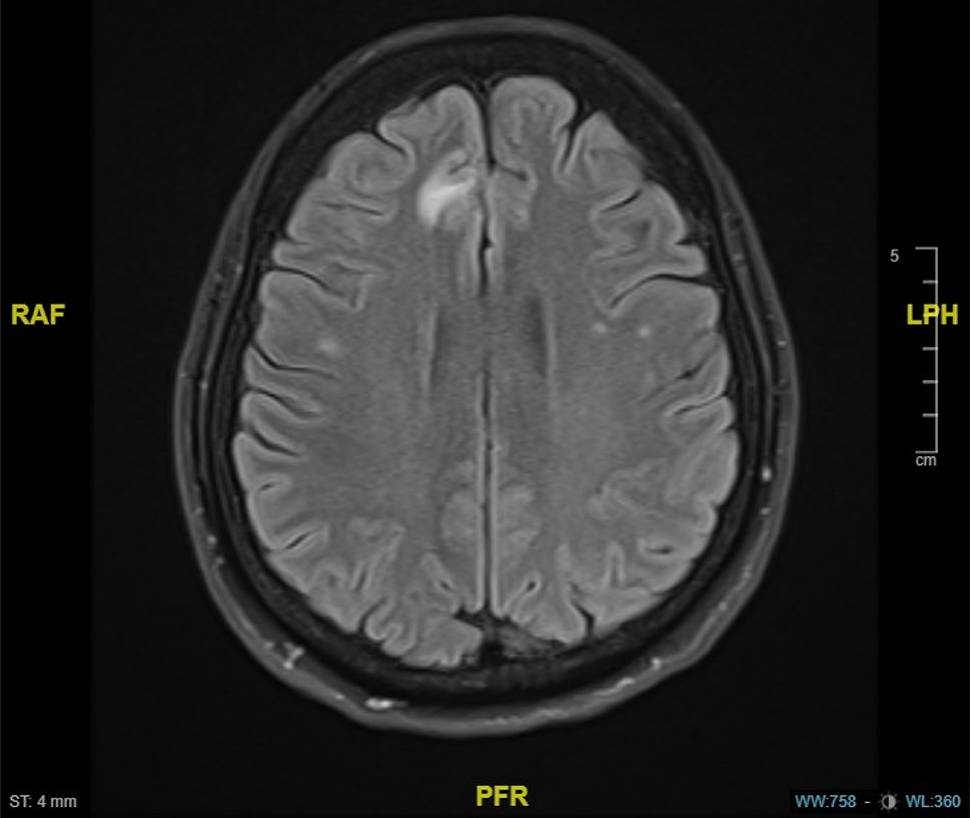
In 2018, the patient was diagnosed with bone metastases in follow-up imaging and therefore denosumab was added as antiresorptive therapy to the therapeutic regime. One year later the patient presented with progressive neurological symptoms including nausea, headaches, balance disorder and visual disturbances. The MRI showed typical signs of LM. No cytological correlate could be found in the subsequently carried out cerebrospinal fluid puncture. As the graphic signs on MRI were corresponding neurological symptoms, LM was assumed according to the EANO-ESMO guideline definition [6]. In further course of disease MR-graphic signs of LM progression were detected. Further liquor punctions were rejected by the patient. Subsequently a radiation of the neuroaxis was performed. While the visceral disease remained stable, therapy with trastuzumab was continued.
